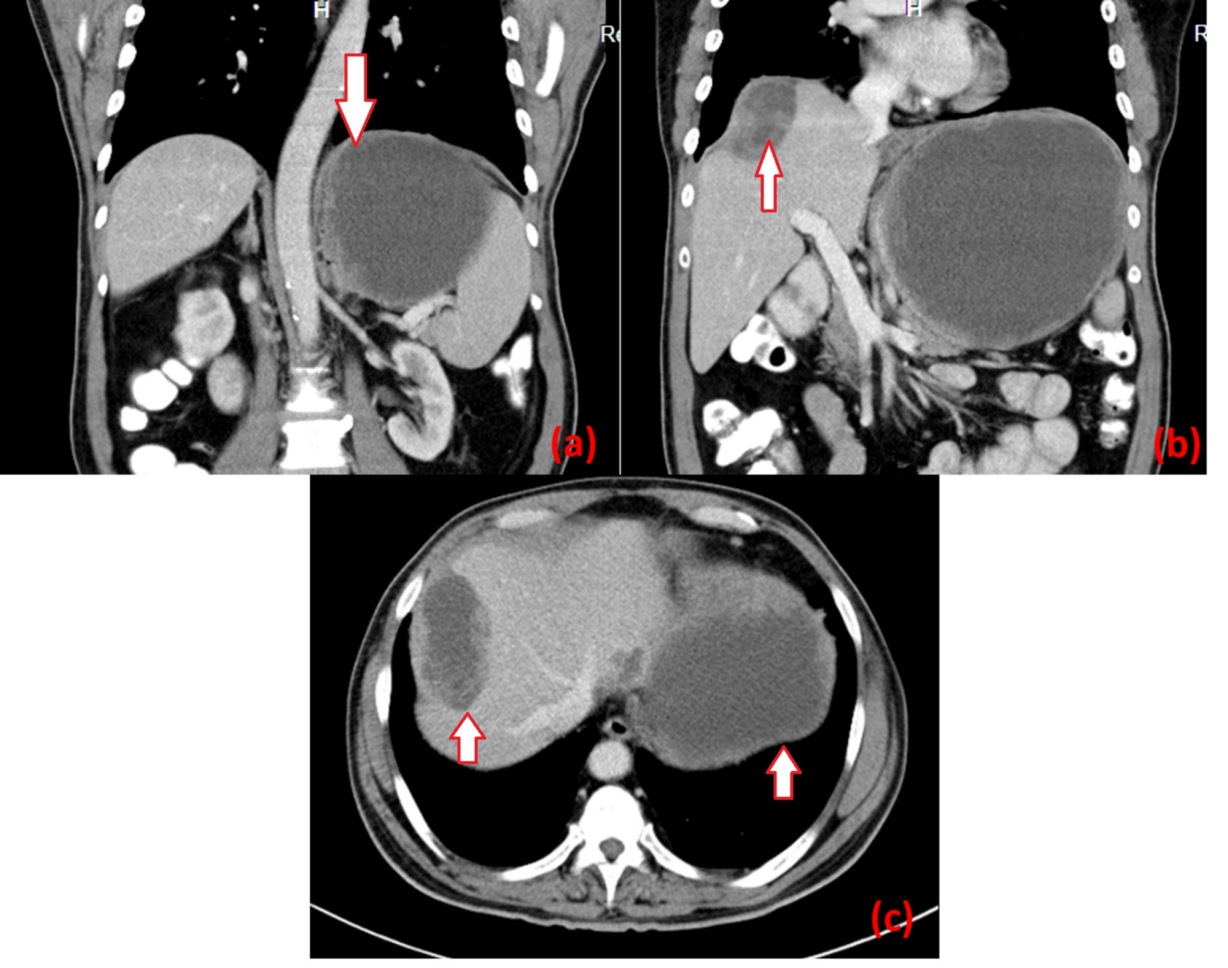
Preoperative CECT images of the abdomen (a) coronal view showing a cystic lesion in the left subphrenic region (arrow); (b) coronal view showing a cystic lesion in segment VII of the liver with thickened wall and few septations (arrow); (c) axial cut showing both liver surface lesion and left subdiaphragmatic lesions (both highlighted by arrows) CECT: contrast-enhanced computed tomography
